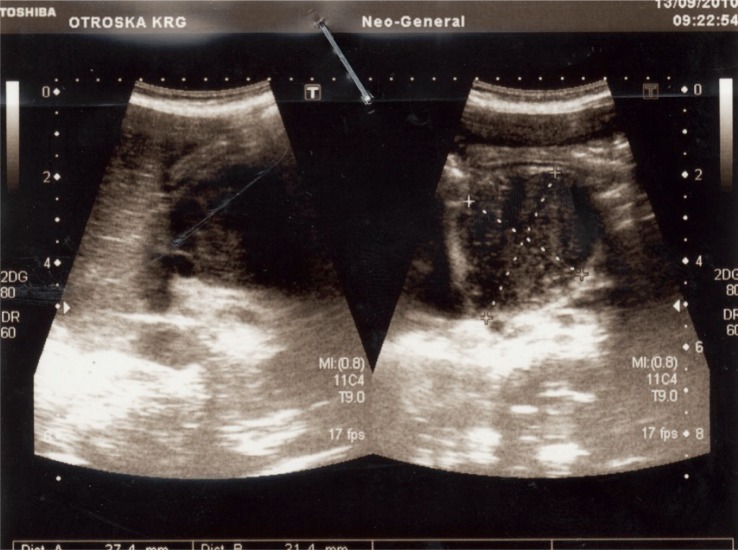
Ultrasound has identified 40 mm large mass in the region of the head of the pancreas.
